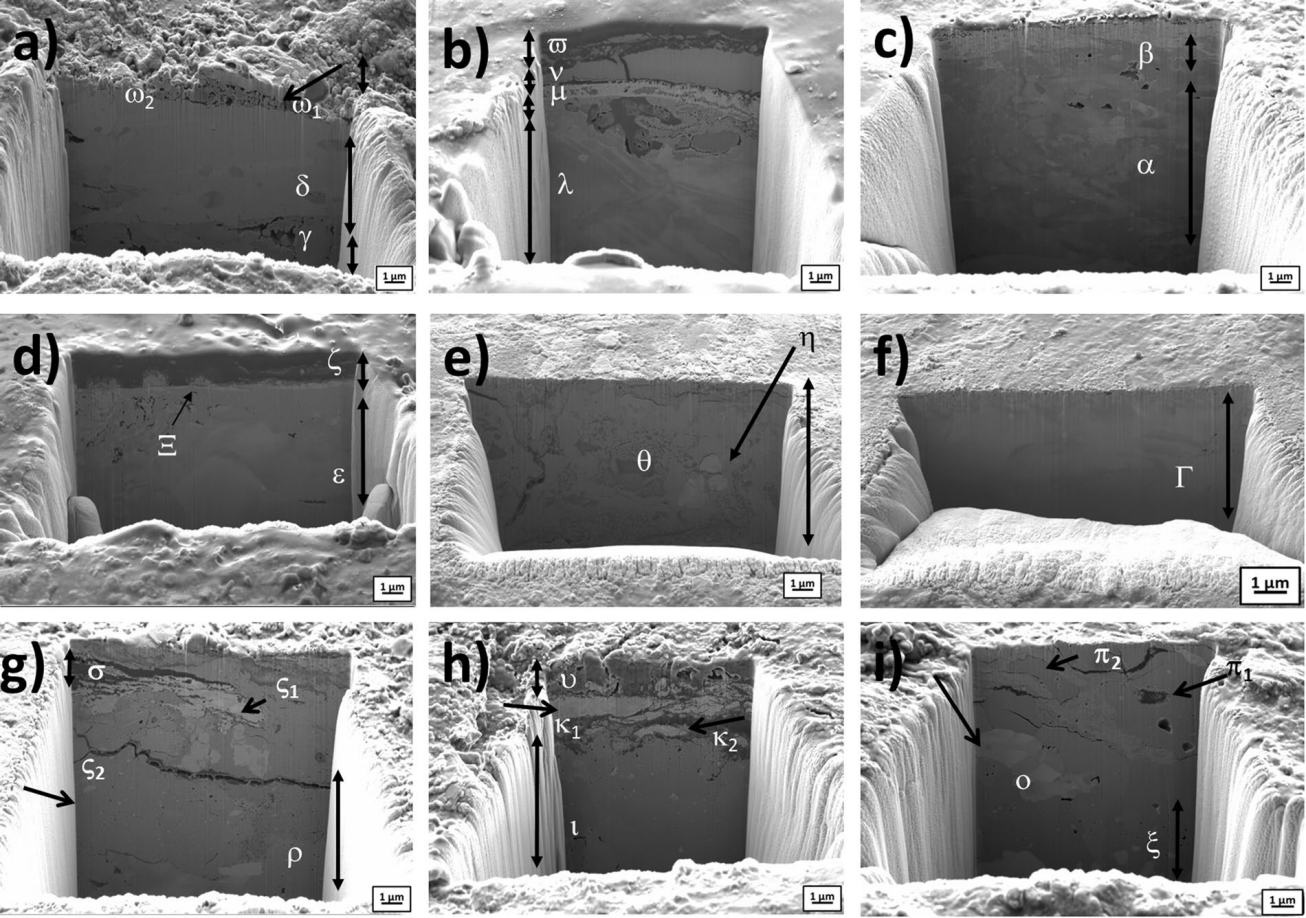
Secondary electron image of the trenches on coins: (a) coin F; (b) coin C; (c) coin A; (d) coin Qui21; (e) coin Pro46; (f) Cla87; (g) coin H, (image width 24 μm); (h) coin B, and (i) coin D. Points in the different layers and phases where X-ray microanalysis was carried out are labelled with Greek characters. Trench image width 15 μm.
